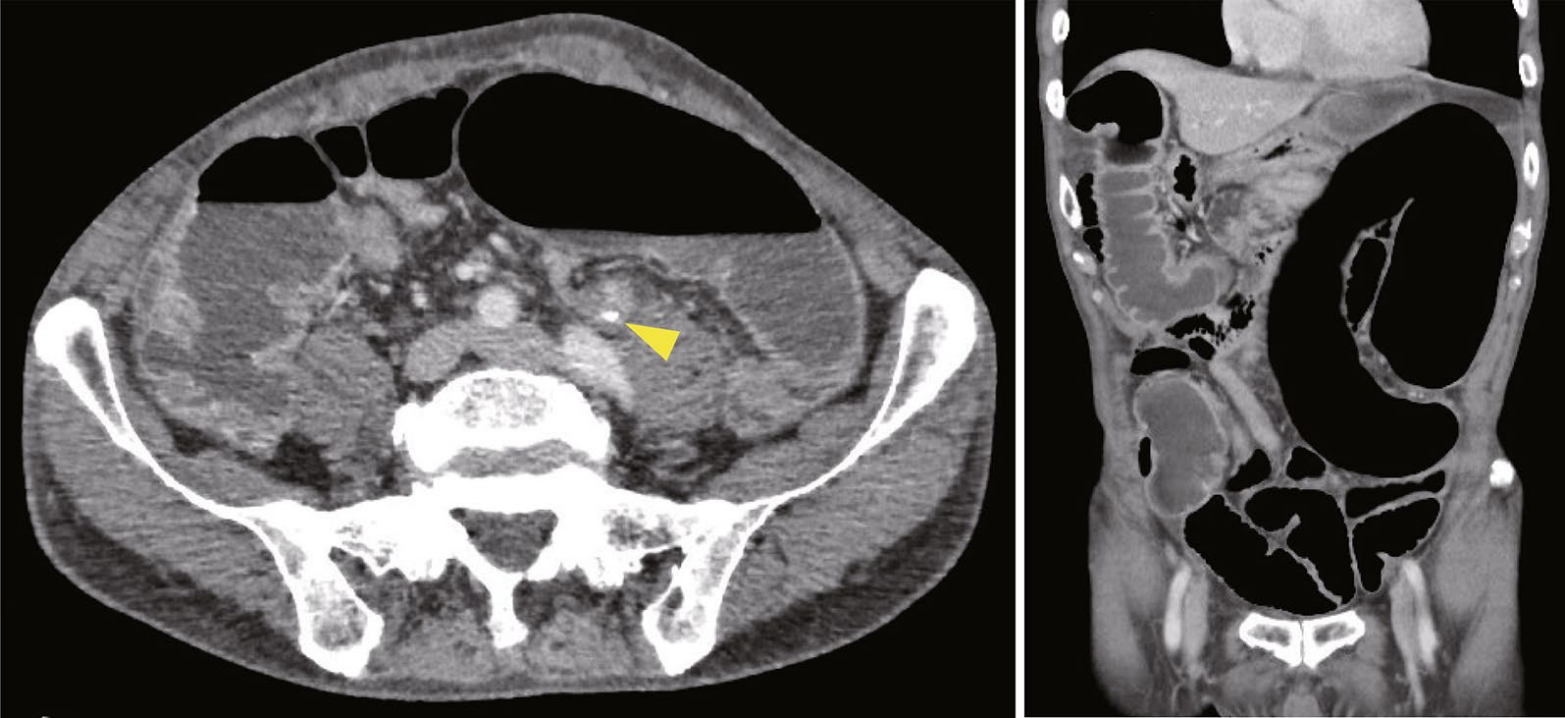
CT images from the time of the onset of sigmoid volvulus demonstrated the sigmoid colon twisted and distended around the anastomotic site. On the left is the axial view and on the right is the coronal view. Yellow arrowheads indicate the previous DST anastomotic site
